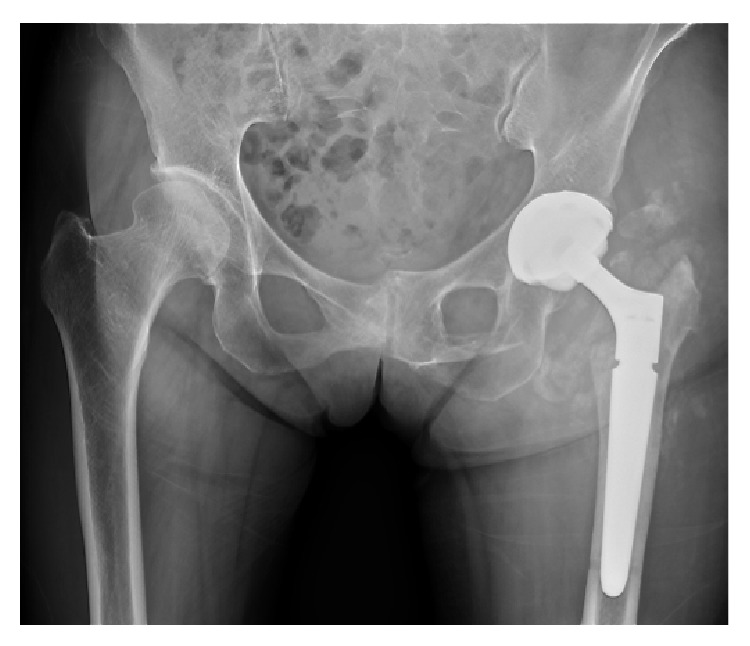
Postoperative radiographs of the left femur showing revision surgery with total hip arthroplasty.
